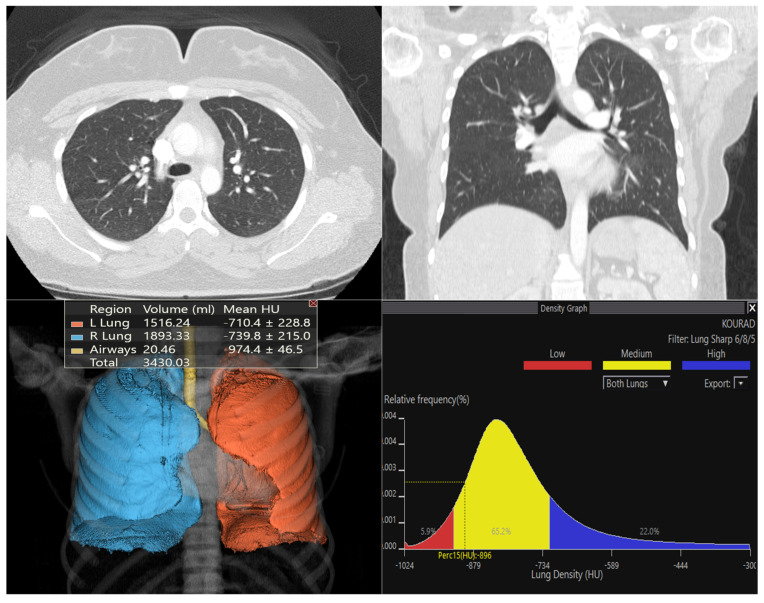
Quantitative analysis of a control subject with no respiratory pathology. The upper panel shows normal parenchymal aeration and vascular structures on axial and coronal computed tomography (CT) sections. The lower left quadrant displays 3-dimensional (3D) volumetric segmentation, where the right (blue) and left (orange) lung volumes were calculated separately (right: ~1893 mL; left: ~1516 mL). The density histogram analysis in the lower right quadrant reveals a normal Gaussian distribution, with mean parenchymal attenuation values ranging from −710 HU to −740 HU, showing no significant deviation indicative of fibrosis (high density) or air trapping (low density).
